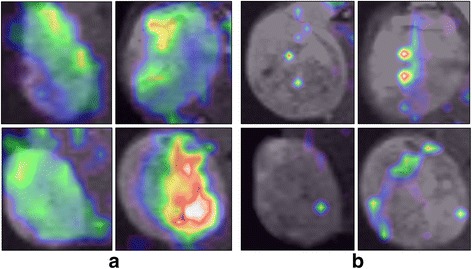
a. Details of xenograft tumours: T2-weighted images fused on corresponding colour-coded apparent diffusion coefficient (ADC) maps. Upper row: tumours from cell line A549; lower row: tumours from cell line H1299. Left column: non-co-transplanted tumours; right column: co-transplanted tumours. Left column: the image fusion of the ADC_diff_ maps of non-co-transplanted tumours reveals comparatively homogenous and intermediate values of diffusion (*gree*n). Right column: the image fusion of the ADC_diff_ maps of co-transplanted tumours reveals the existence of extreme values of diffusion (*red* = high; *dark blue* = low). Range of ADC_diff_ (mm^2^/s × 10^−6^) in the tumour were: top line left, 312–1593; top line right, 31–1586; lower line left, 55–1920; lower line right, 30–2584. b. Details of xenograft tumours: T2-weighted images fused on corresponding colour-coded ADC maps. Upper row: tumours from cell line A549; lower row: tumours from cell line H1299. Left column: non-co-transplanted tumours; right column: co-transplanted tumours. Left column: the image fusion of the ADC_perf_ maps of non-co-transplanted tumours reveals only very few vessels that are large enough to be visualised (*green dot*). Right column: the image fusion of the ADC_perf_ maps of co-transplanted tumours reveals larger perfused vessels (*green linear structures*). Range of ADC_perf_ (mm^2^/s × 10^−6^) in the tumour were: top line left, 3–3502; top line right, 1–3977; lower line left, 1–2733; lower line right, 2–3920
